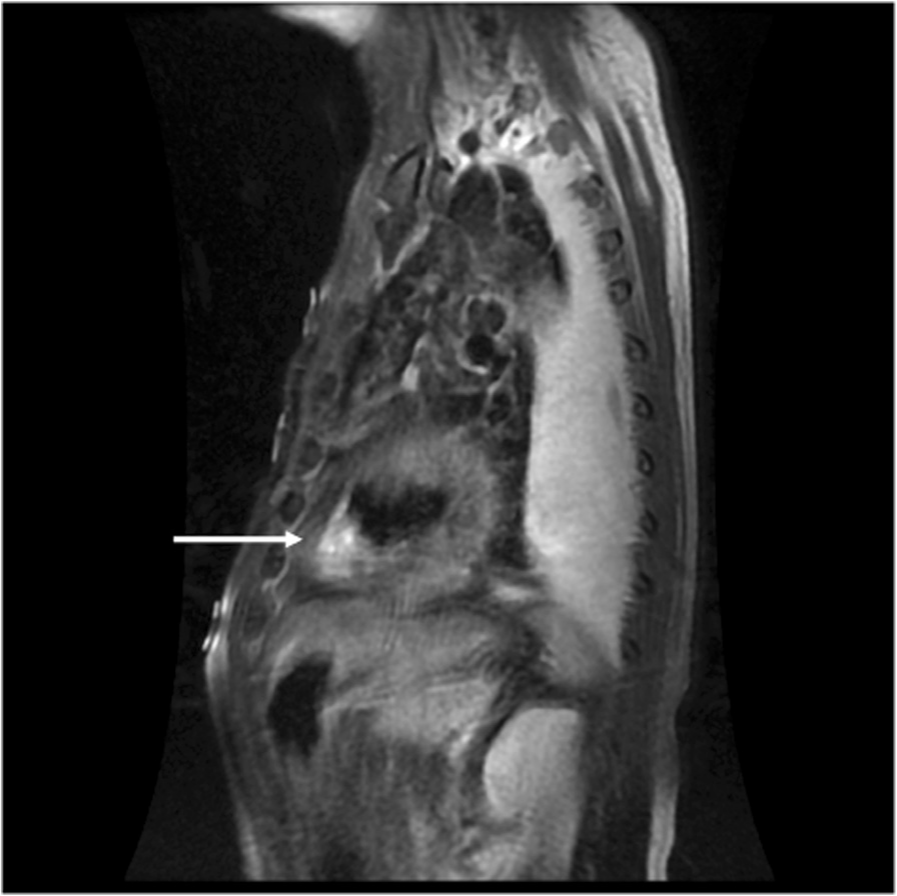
T2-weighted contrast-enhanced MRI view revealed gadolinium enhancement in the left ventricular wall, especially at the anterior-inferior wall of the left ventricle, consistent with myocarditis (a)
